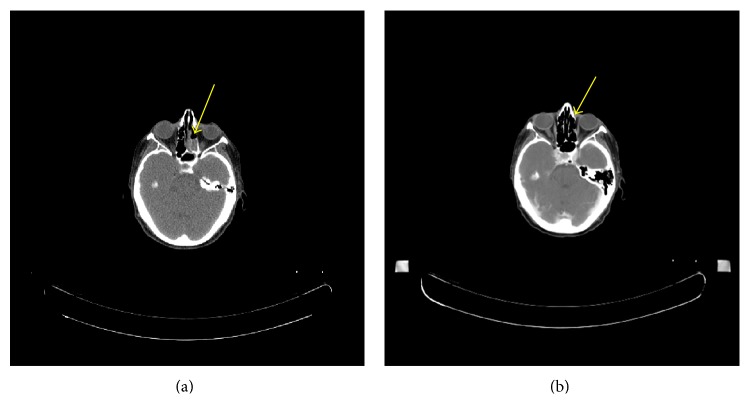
Images from computed tomography scanning prior to starting chemotherapy and after three cycles, showing resolution of left nasopharyngeal mass.
